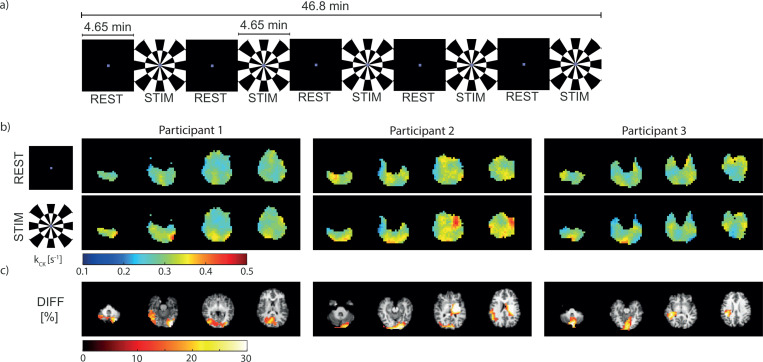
(a) The visual stimulation paradigm consists of 5 interleaved blocks of rest (REST) and stimulation (STIM). Each block takes 4.65 min, resulting in a total duration of 46.8 min. (b) In vivo brain $k_{\text{CK}}$ maps estimated from the averaged REST and STIM blocks, respectively, and (c) the percentage difference change (DIFF) from REST to STIM. Clusters (size ≥ 20) with a change ≥ 10% are displayed. The DIFF map is overlaid on a coregistered $T_{1}$-weighted ^1^H-MR image.
